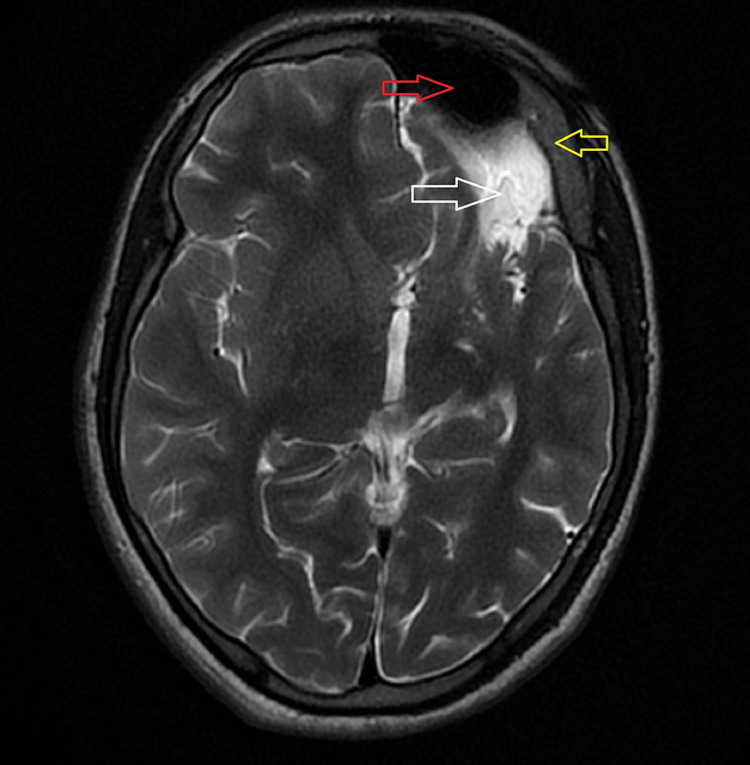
Axial T2 weighted MRI of the brain showing volume loss involving the left cerebral hemisphere (white arrow); Ipsilateral calvarial thickening (yellow arrow); Hyperpneumatization with dilatation of frontal sinus (red arrow). MRI: magnetic resonance imaging
